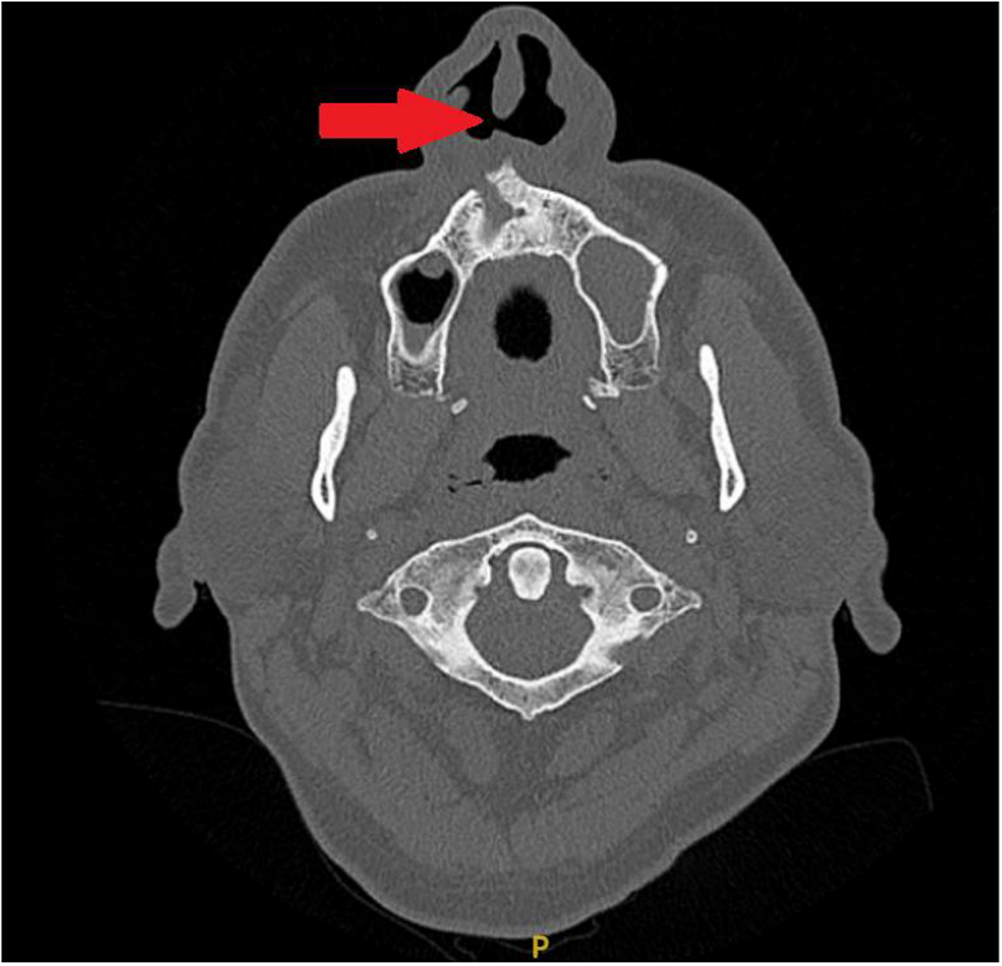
CT sinus (axial view), with the red arrow showing nasal perforation.
